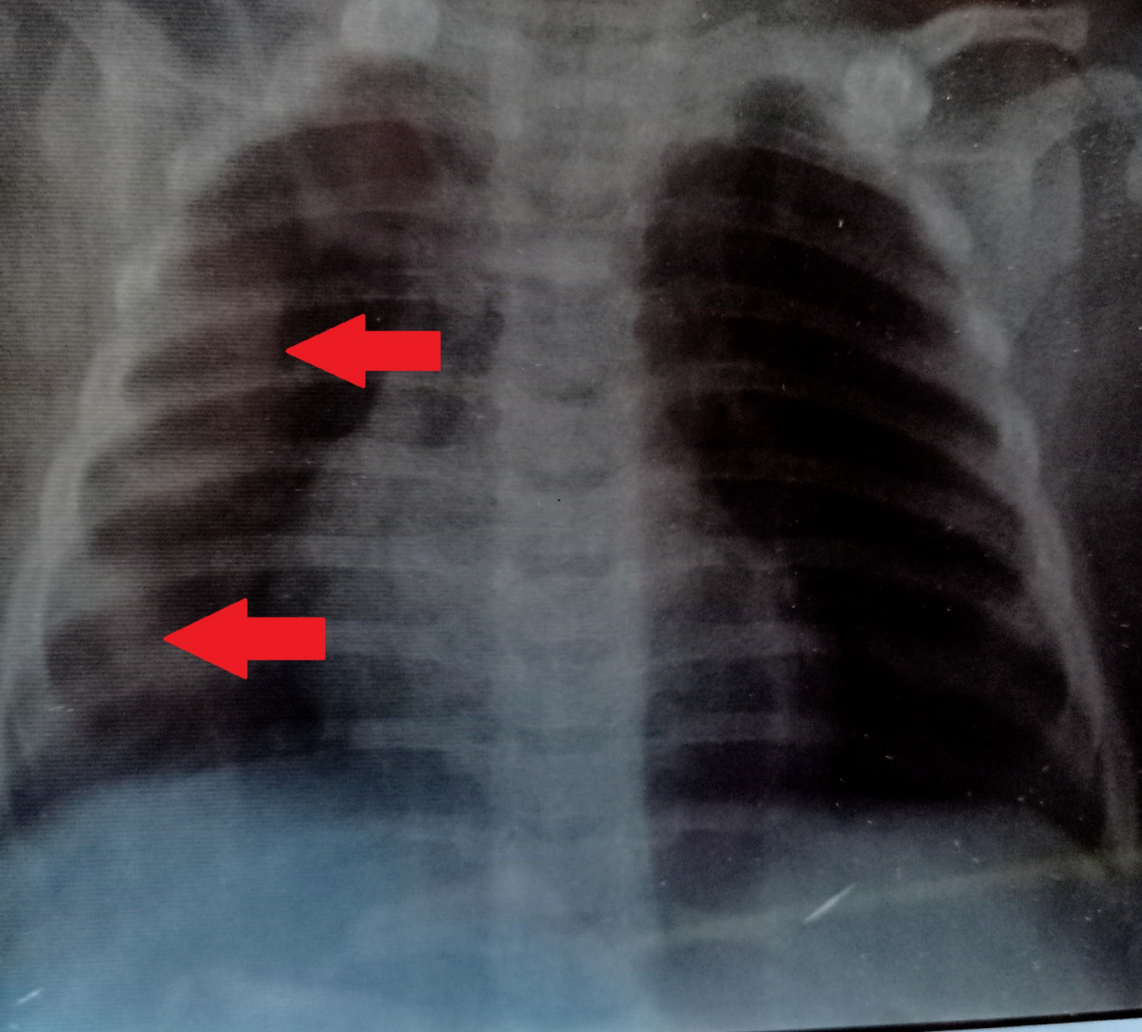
Chest X-ray revealing haziness in the right middle and lower lobes (red arrows).
